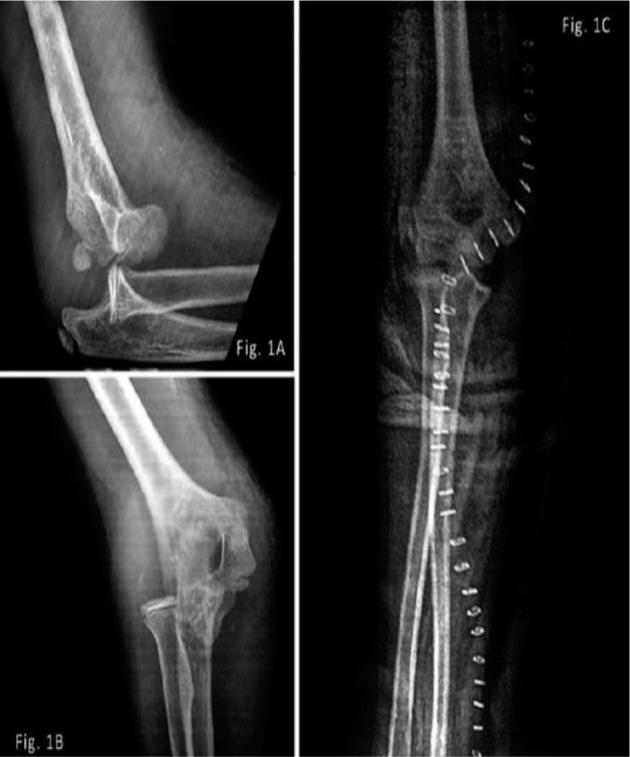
1A, 1B showing posteromedial dislocation of the elbow Fig. 1C post-reduction radiograph showing curvilinear incision closed with staples.
